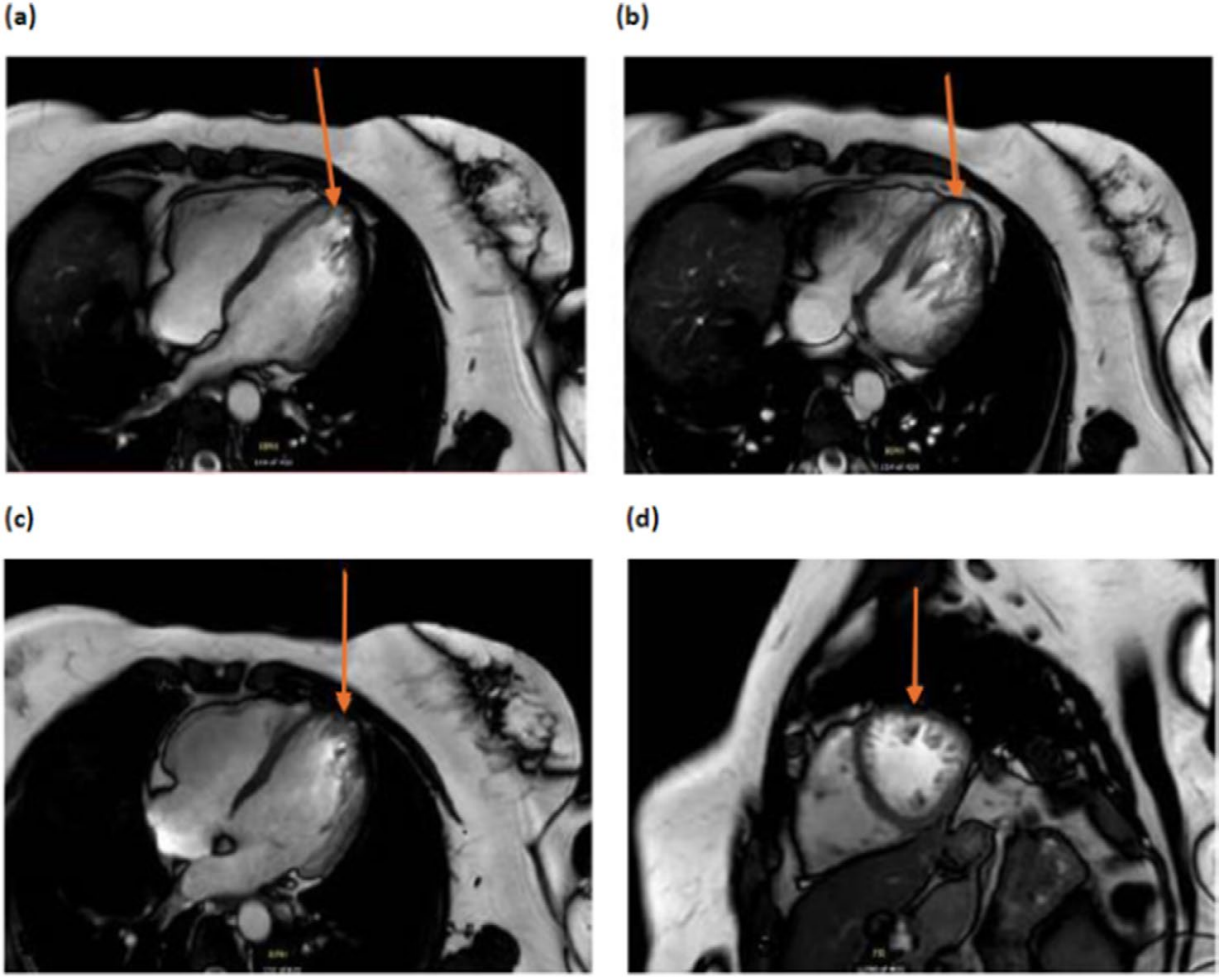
(a–c) Showing MRI coronal views of the left ventricle, (d) sagittal view of the left ventricle showing prominent apical trabeculations.
